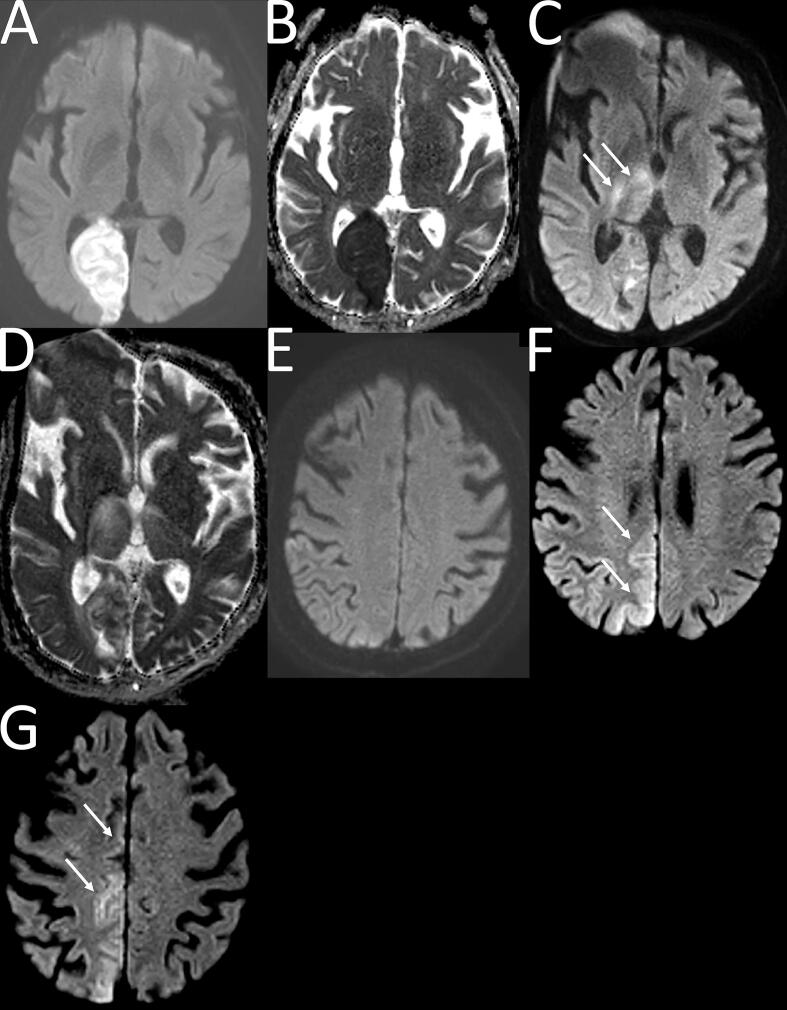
Panel of MRI scans of the brain. Legend: 1A – 1B show an acute right occipital lobe infarct. 1A = MRI Diffusion-weighted b1000 image and 1B = ADC map. Taken together, they show marked diffusion restriction in the right occipital lobe, in keeping with an acute infarct. 1C – 1E show MRIs following patient’s admission with confusion and seizures. 1C = Diffusion-weighted b1000 image and 1D = ADC map. Taken together, they show significant resolution of the acute changes, as compared to in 1A – 1B. Changes in the right thalamus (white arrows), not present previously, are likely due to progression of ischaemia. Distortion in the right frontal lobe is artefactual (due to patient movement). 1E is a diffusion weighted b1000 image which, at this stage, does not show any change in the right medial parietal cortex or the cingulate gyrus. 1F – 1G show the MRIs taken a few weeks later. Patient continued to have focal motor seizures. 1F and 1G are diffusion-weighted b1000 images showing a degree of cortical based/gyriform/ ‘ribbon-like’ diffusion restriction (ADC map not shown) in the right medial parietal cortex and extending anteriorly along the cingulate gyrus, not present previously (compared to 1E). Excitotoxicity due to seizure-related activity and extension of ischaemia are possible causes. Given the contiguous cortical involvement of both anterior and posterior circulation territories in the context of on-going seizures, seizure-related changes are favoured.
